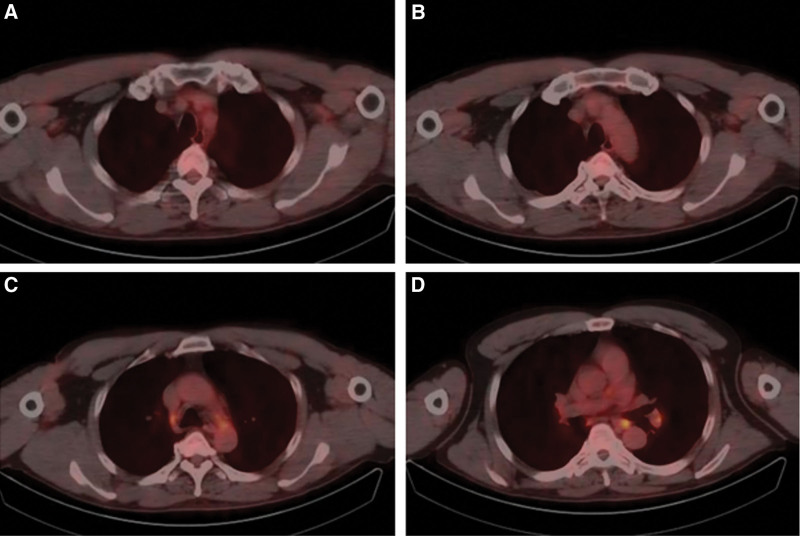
18F-FDG-PET imaging in September 2021 demonstrating slight hypermetabolic mediastinal and bilateral hilar lymph nodes. FDG = fluorodeoxyglucose, PET = positron emission tomography.
